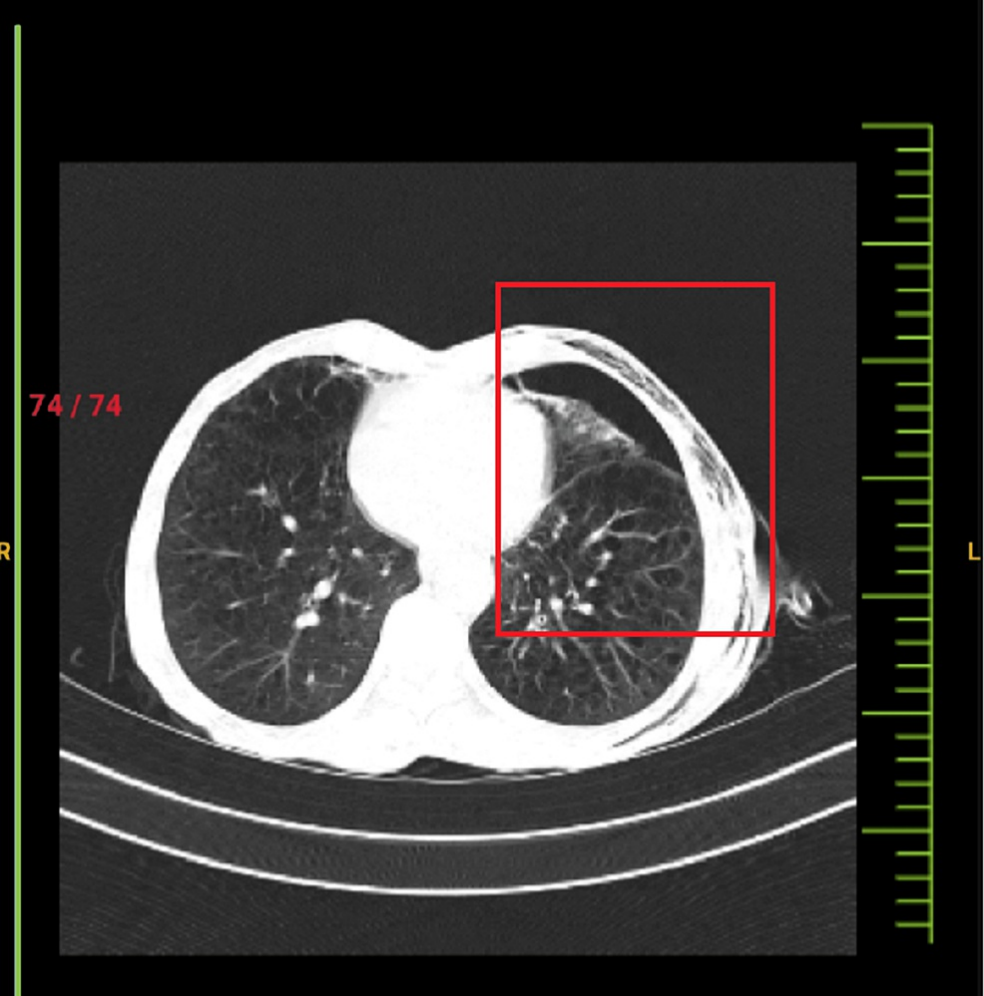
CT Thorax presenting pneumothorax with underlying lung atelectasis
